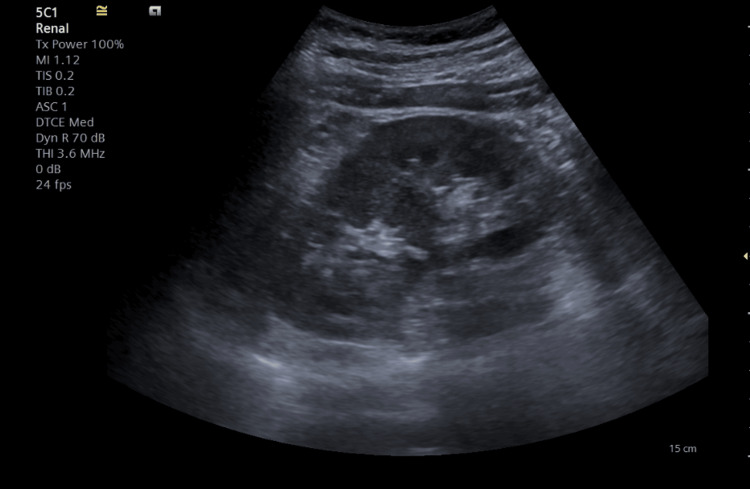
Ultrasound image demonstrating the absence of urinary extravasation
